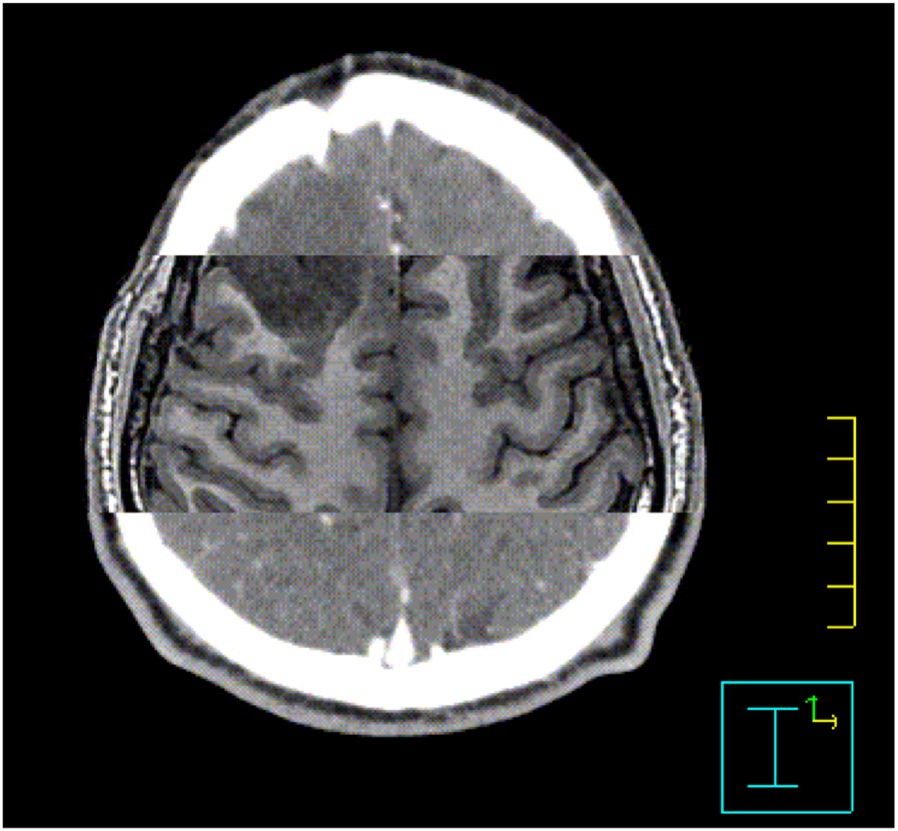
T1-weighted MR imaging and the corresponding axial CT after registration.
